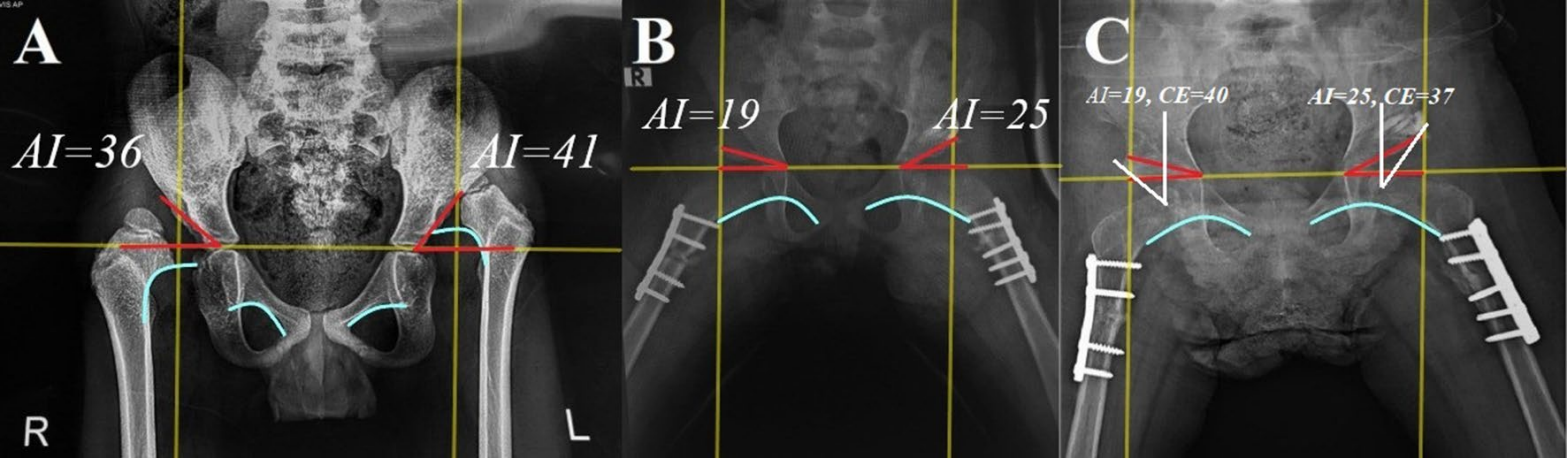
Male child, 72 months, presented with bilateral DDH, Tönnis grade III on the right side and grade IV on the left side. A preoperative. B Immediate postoperative. C After 18 months of follow-up the radiographic result was Severin’s grade I on the right side and grade II on the left side
